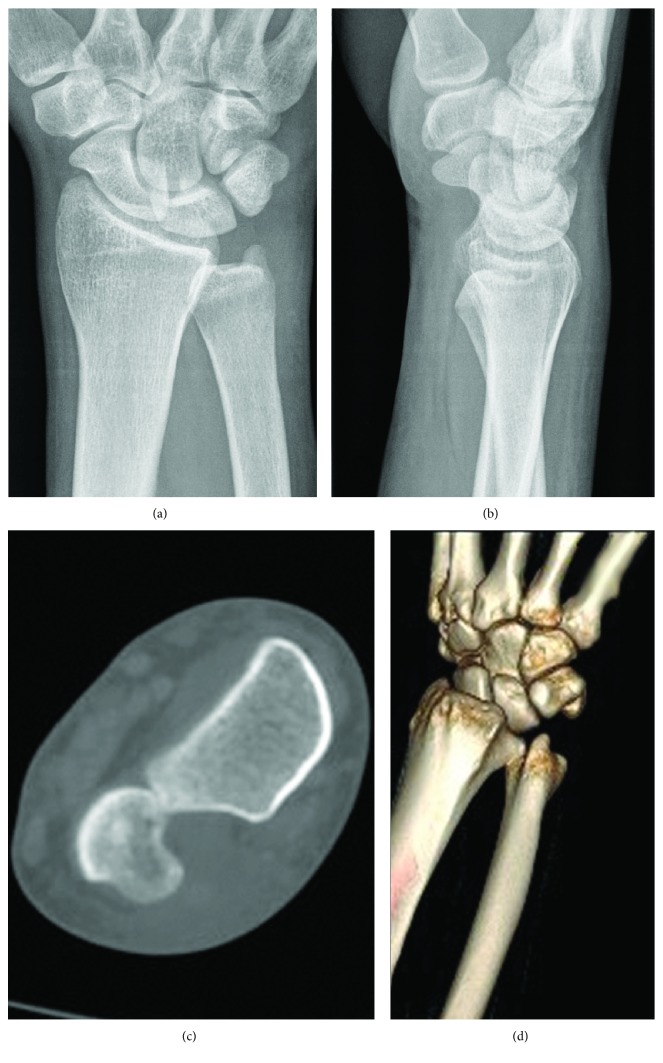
AP (a) and lateral (b) radiographs of the injured wrist. Notice overlap between the radius and ulna on the AP view and slight anterior position of the ulna in relation to the carpal bones on the lateral view. Select coronal cut (c) and 3D reconstruction (d) of the forearm clearly show that the ulnar head is dislocated outside of the sigmoid notch and impacted on the radius.
